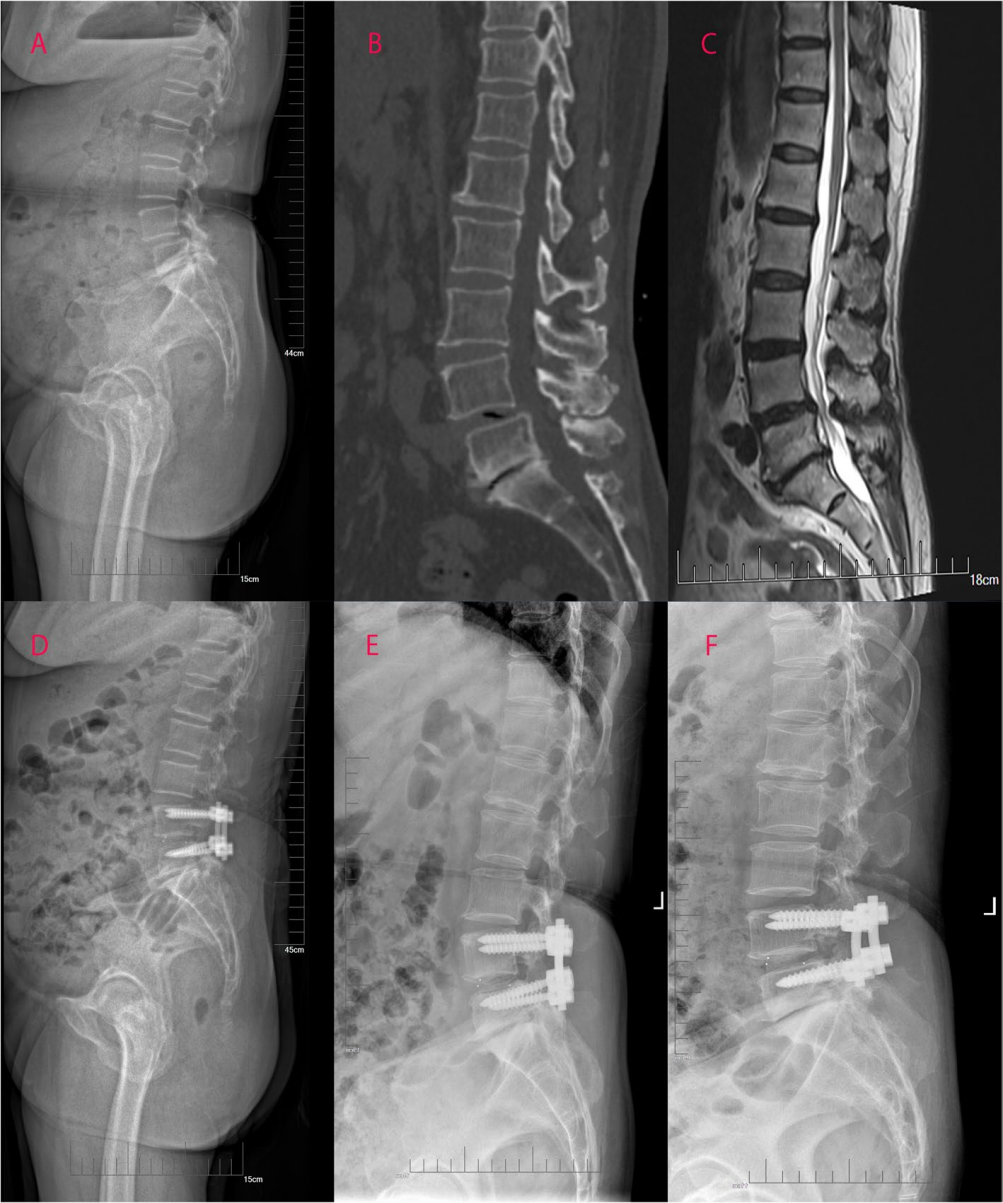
Preoperative sagittal lateral view (A), sagittal-computed tomographic scan (B), sagittal T2-weighted magnetic resonance image (C), sagittal lateral view one month after surgery (D and E) and sagittal lateral view at the final follow-up (F) of a 67-year-old female patient with L4 degenerative spondylolisthesis treated with PLIF with long screw fixation
